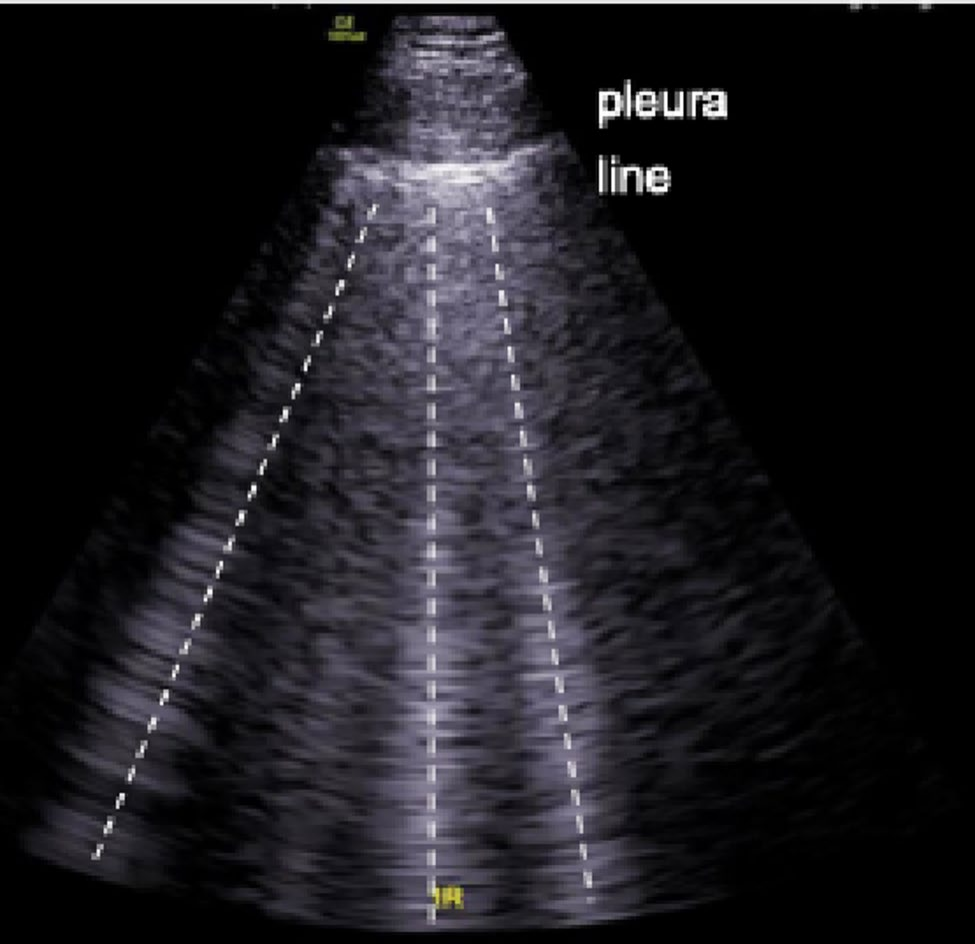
Ultrasound image of B-lines. Dashed lines represent “comet tail” artifact
